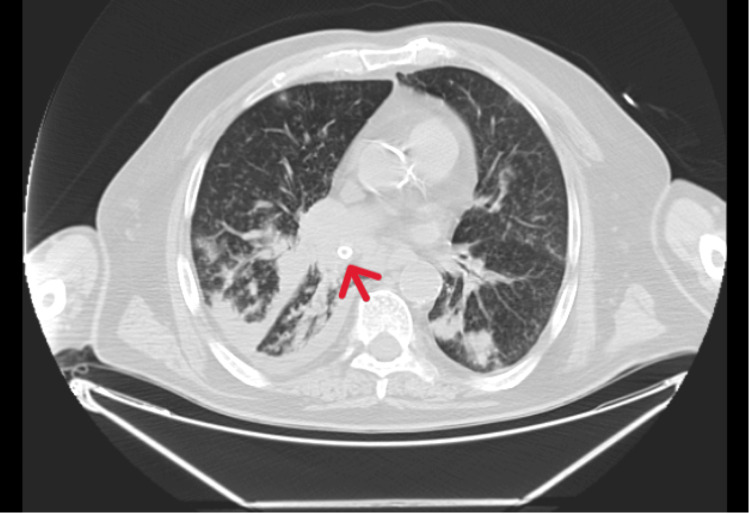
Chest CT scan showing mucous filling of the bronchial tree in the basal segments of the right lower lobe, indicating the presence of an oval structure with coarse peripheral calcification (red arrow), apparently in an intraluminal location in the respective lobar bronchus with subtotal pulmonary atelectasis of this lung lobe
